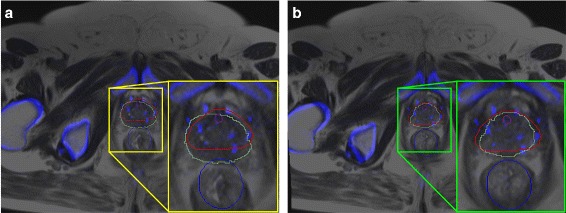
Qualitative results for multi-modal registration a rigid registration, b deformable registration. The high contrast structures from CT (*blue shade*) are overlaid onto MRI. It can be observed that the bone structures are aligned with a rigid registration alone. A deformable registration around the prostate region helps align the prostate (*red contour* on CT and *green contour* on MRI) after deformable registration. The prostate region is zoomed in and shown in the inset
